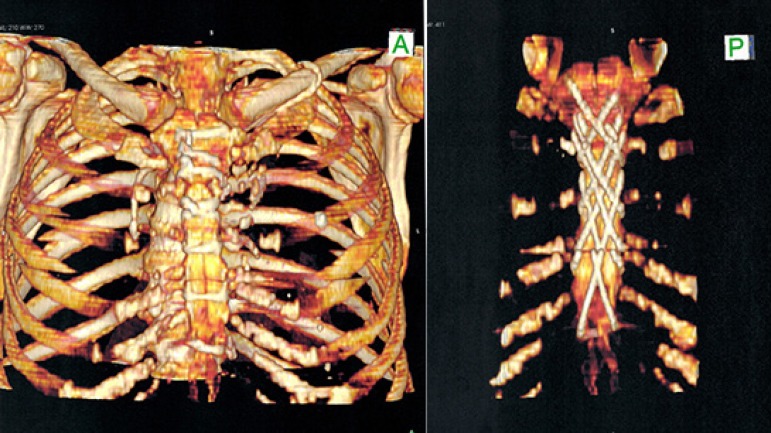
Chest computed tomography showing (A) anterior and (P) posterior
